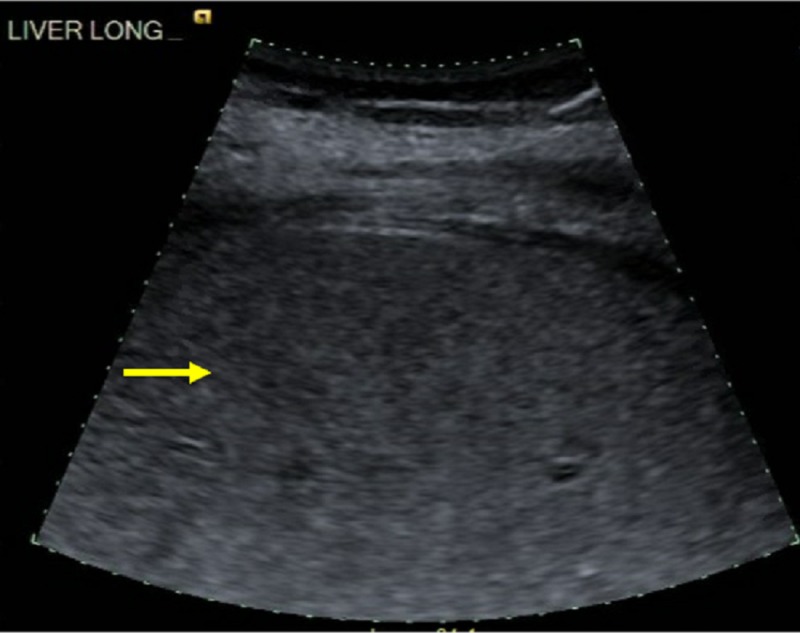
Ultrasound abdomen liver long axis showing steatosis
